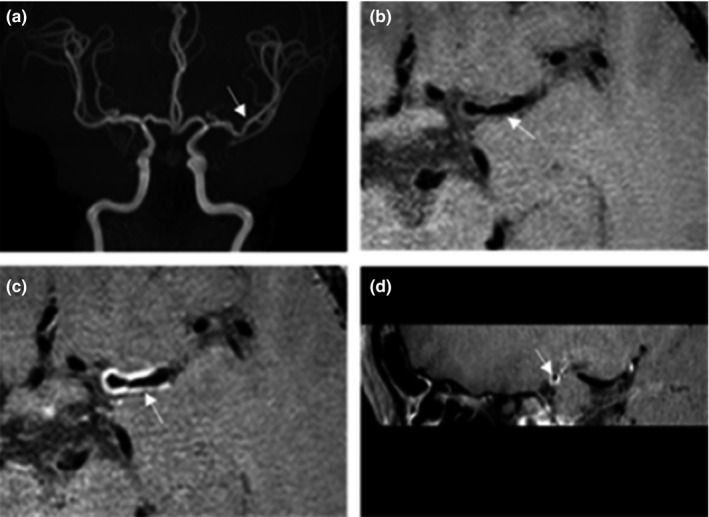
(a) Male, 33 years old, 3D‐TOF MRA showed a slight decrease in the signal of the left middle cerebral artery, (b–d) HR‐MRI shows segmental uniform thickening and strengthening of the wall, and the lumen was estimated to be a mild stenosis
